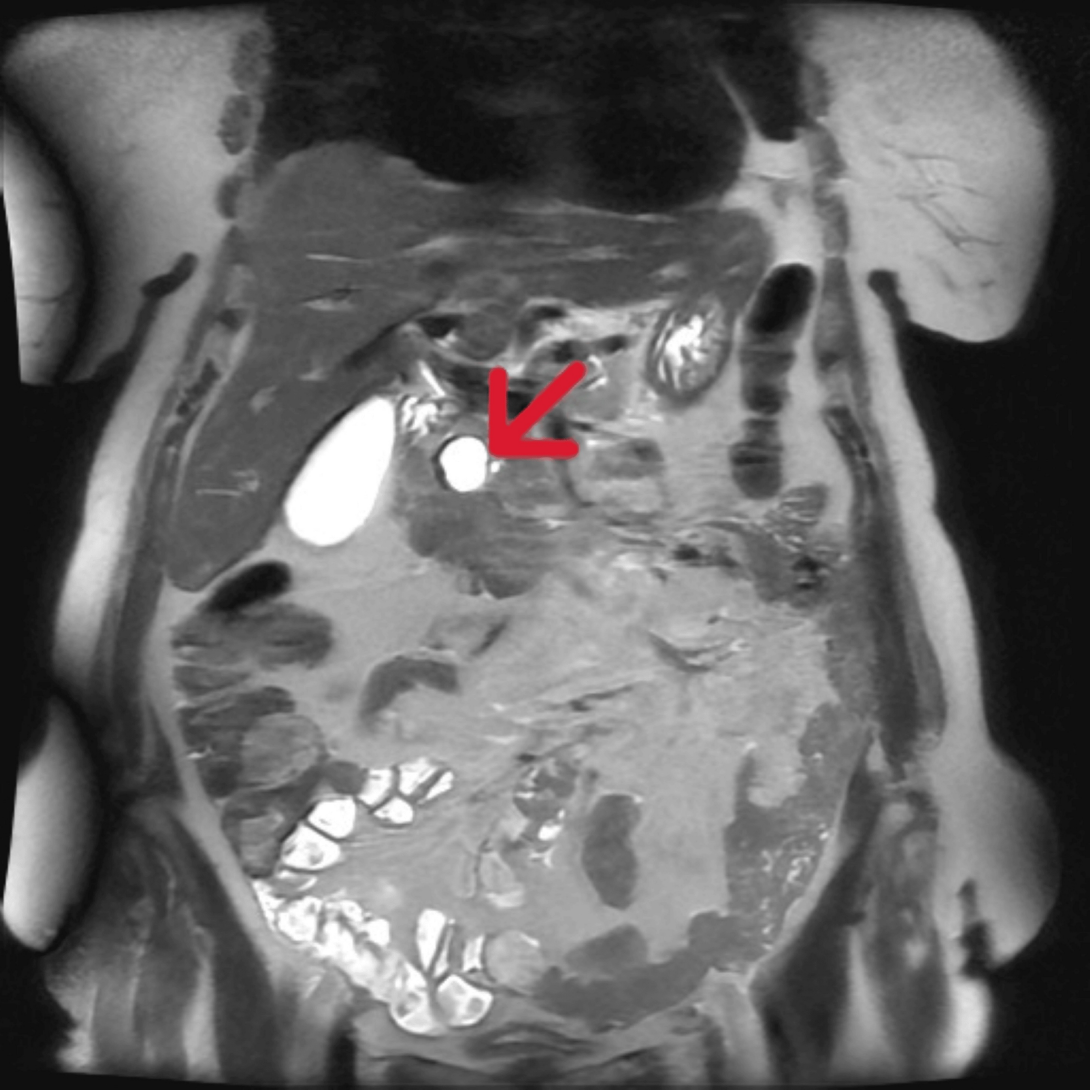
Coronal MRI T2-weighted SSFSE sequence of the abdomen. The image shows a well-defined cystic lesion in the pancreatic head (arrow). SSFSE: single-shot fast spin-echo
